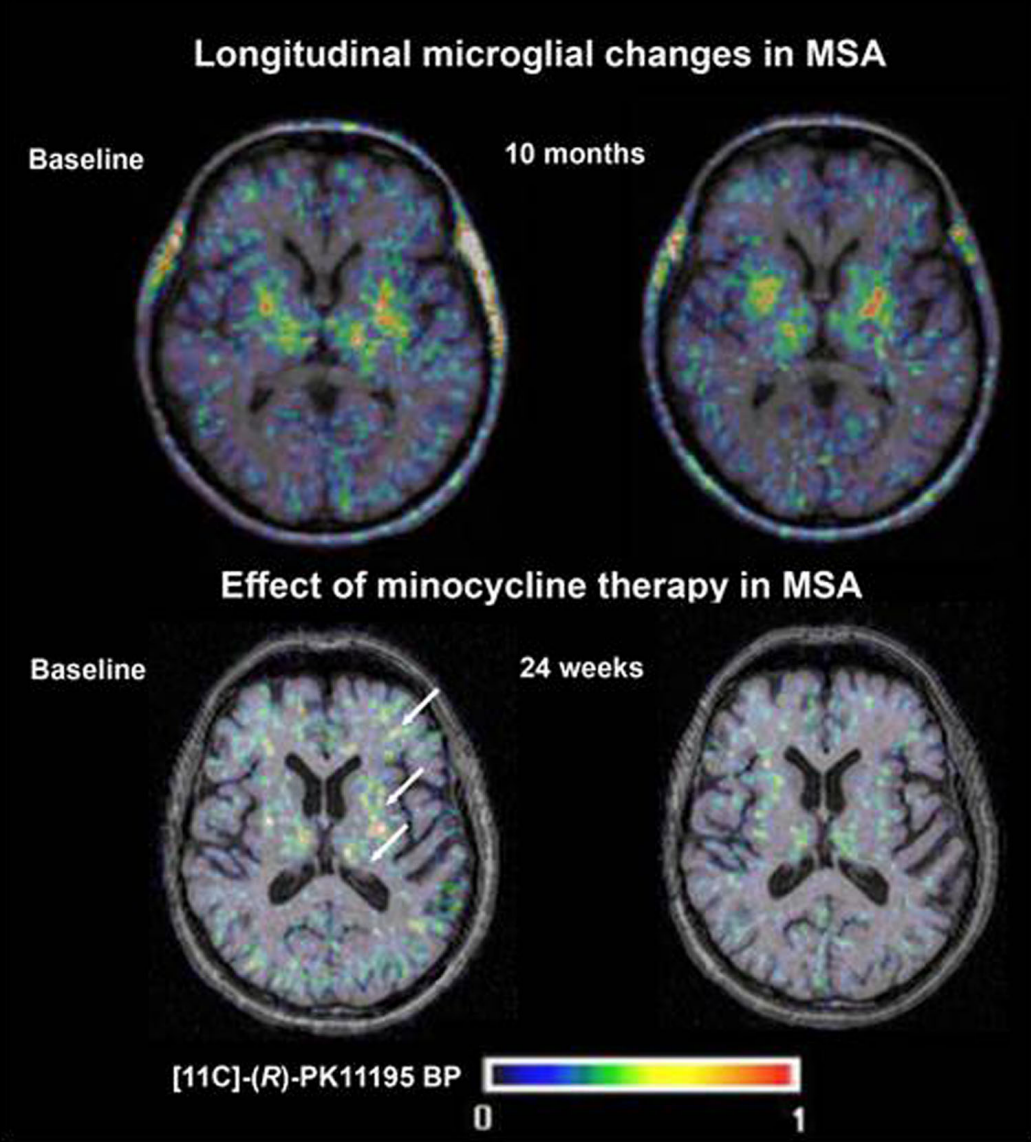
Longitudinal changes in [^11^C]-(*R*)-PK11195 binding potential (BP) seen in a study of minocycline therapy in patients with multiple system atrophy (MSA). Images are transverse BP maps coregistered to structural T1-weighted MR images at baseline (a) and after 24 weeks of treatment (b). Modified with permission from [37]
